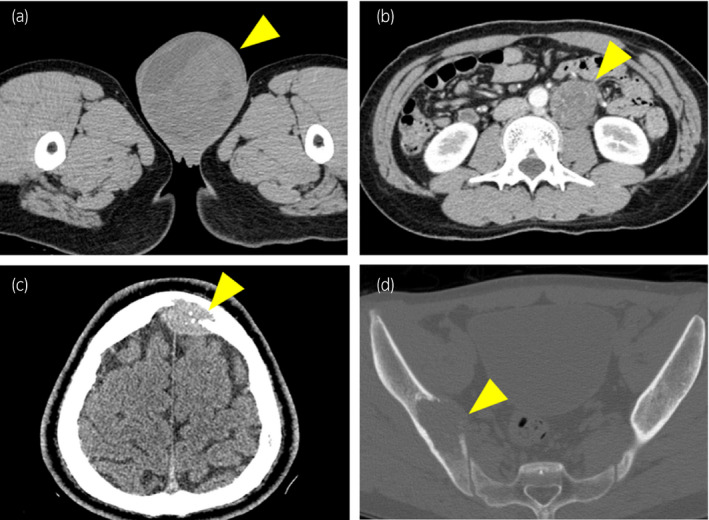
CT revealed a left testicular tumor and metastatic tumors. (a) A large left testicular tumor of 14 × 9 cm was observed in the testis (arrowhead). (b) An enlarged para‐aortic lymph node was evident (arrowhead). (c) A metastatic tumor with bone destruction was identified in the frontal bone (arrowhead). (d) An osteolytic tumor was observed in the left ilium (arrowhead).
